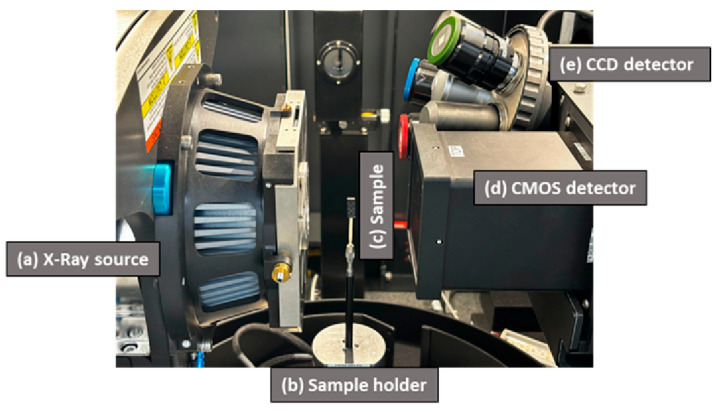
Zeiss Xradia versa 515 imaging system with sub-micron resolution used in the present study. The system consists of (a) an X-ray source [Polychromatic source with 160 kV tube voltage and 10 W power output], (b) a rotating sample holder, (c) a sample mounted on the sample holder, (d) a CMOS Flat Panel Detector- ideal for large field-of-view imaging and (e) a CCD Detector: having magnification options of 0.4×, 4×, 20×, and 40× for high-resolution studies across scales.
